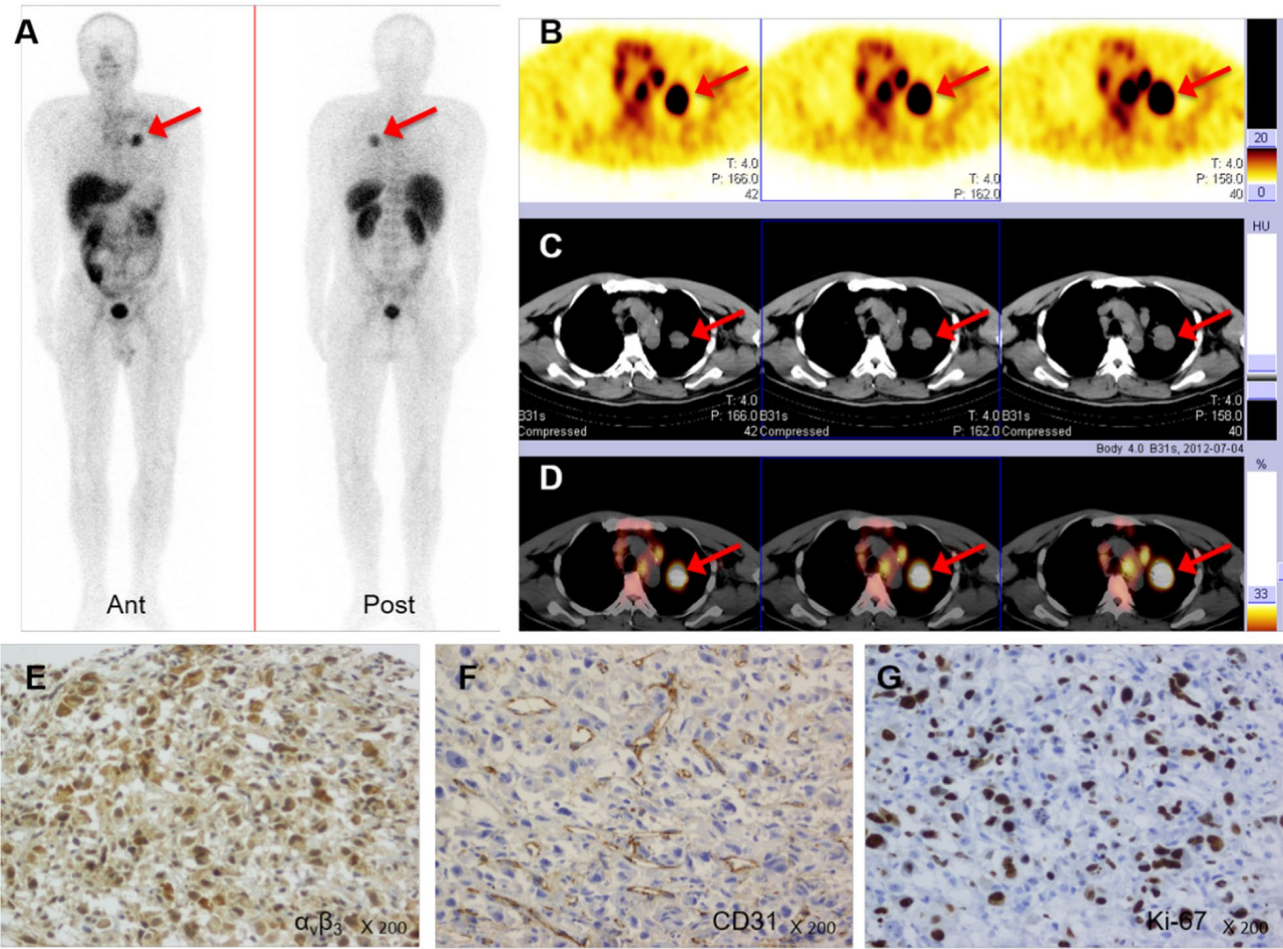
[^99m^Tc]Tc-Galacto-RGD_2_ SPECT/CT detected the primary tumour and multiple lymph node metastases in advanced adenocarcinoma. [^99m^Tc]Tc-Galacto-RGD_2_ image (a–d). a Whole-body image. b–d SPECT/CT. e–g IHC showed higher expression of α_v_β_3_ in the tumour cells and neo-vasculature, higher density of micro-vessel with CD31 expression in the tumour tissue, and a higher Ki-67 index
